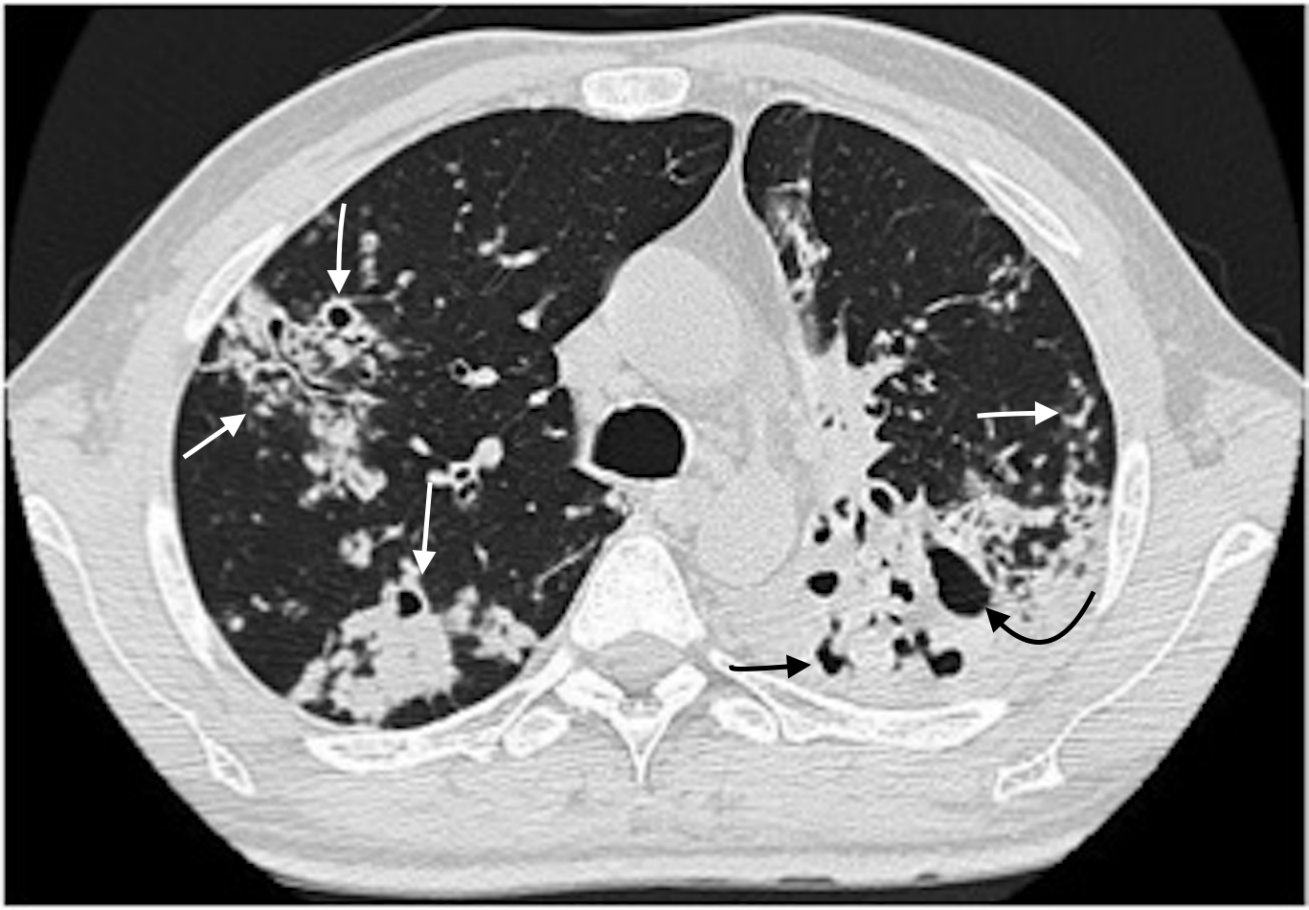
Primary multidrug-resistant TB in a 39-year-old man. Lung window of a transverse thin-section CT scan (1.25-mm-section thickness) showing multiple consolidations, small centrilobular nodules, tree-in-buds and bronchiectasis (arrow). A cavity in consolidation at the left lower lobe was also noted (curved arrow).
